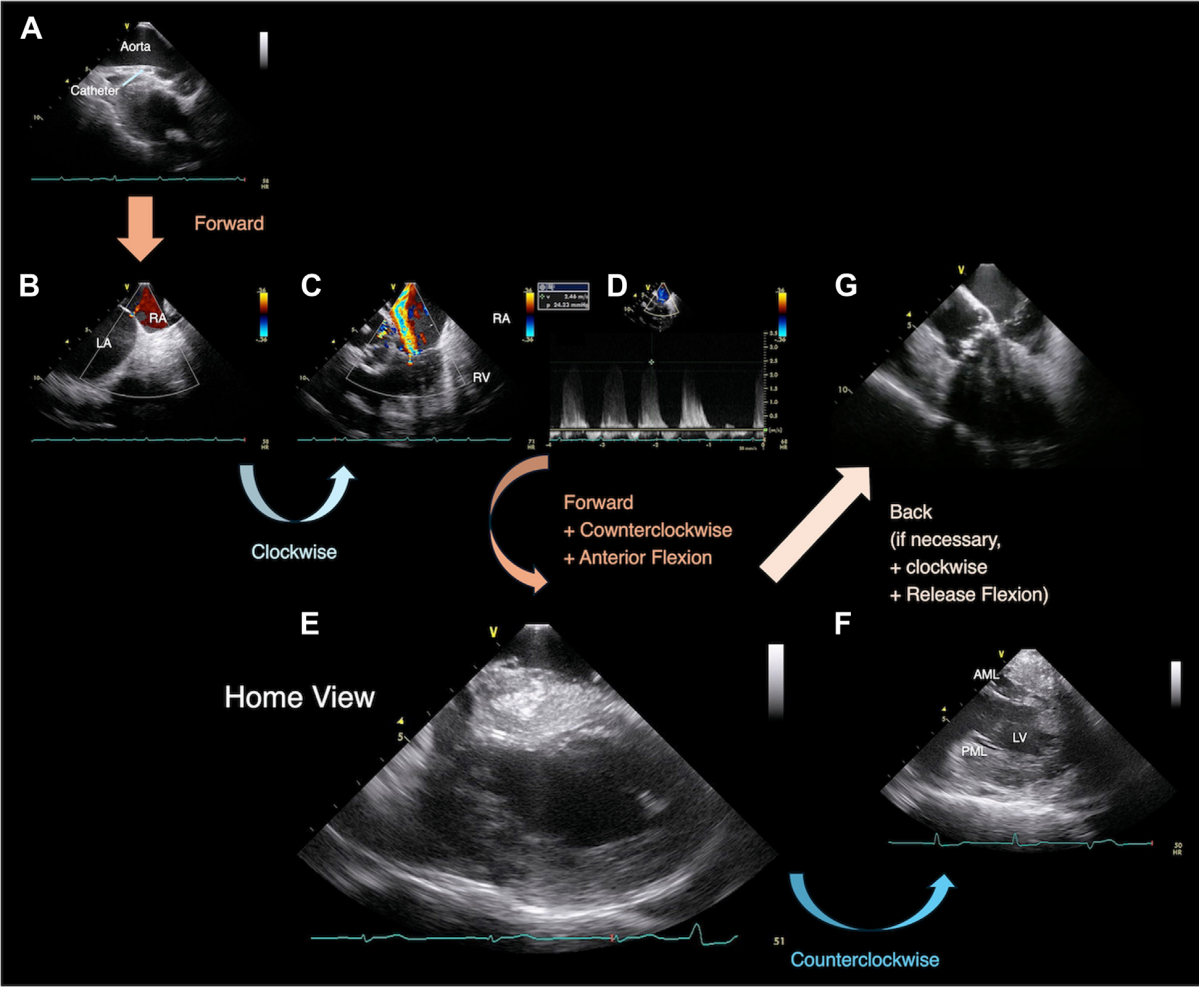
Multiple Views With Jugular Vein Intracardiac Echocardiography Identifying Complications or Hemodynamics (A) Long-axis ascending view. (B) Atrial septum view. There is small atrial septal defect with left-to-right shunt flow. (C) Long-axis tricuspid view. There is mild to moderate tricuspid regurgitation. (D) Pulse Doppler image of tricuspid regurgitation demonstrating the pressure gradient from the right ventricle (RV) to the right atrium (RA) of 24 mm Hg. (E) Long-axis left ventricular view. (F) Long-axis mitral valve view. (G) Long-axis aortic valve view. AML = anterior mitral leaflet; LA = left atrium; LV = left ventricle; PML = posterior mitral leaflet.ii.Atrial septum view (Figure 7B):•Advance the catheter a few centimeters farther (if necessary, add clockwise rotation).•Check for atrial septal defects or a patent foramen ovale.iii.Long-axis right ventricular (RV) view (Figure 7C):•Rotate the catheter clockwise.•Assess for preoperative tricuspid regurgitation and estimate RV systolic pressure (Figure 6D).iv.Long-axis LV view (home view) (Figure 7E):•Push forward into the right ventricle with anterior flexion and counterclockwise rotation.•Evaluate LV contraction, pericardial effusion, and wire position during the procedure.v.Long-axis mitral valve view (Figure 7F):•Rotate the catheter counterclockwise.•Assess the mitral valve complex.vi.Long-axis aortic valve view (Figure 7G):•Return the catheter clockwise and pull back to the right atrium.•Evaluate the aortic valve complex (if necessary).•Measure preprocedural aortic valve flow velocity (if necessary).c.Perform pretreatment TTE evaluation.4.Target identification and contrast agent injectiona.Administer analgesics.b.Perform coronary angiography.c.Monitor the contrast injection with ICE.d.Confirm the target location with ICE, and reassess if needed.5.Septal ablation and monitoringa.Continuously monitor with ICE during the ethanol injection.b.Evaluate for complications:i.Wall motion abnormalitiesii.Pericardial effusioniii.Mitral valve function changesiv.Ventricular septal rupturev.Other mechanical complications6.Postprocedure evaluation and managementa.Confirm full-cover ablation using ICE and TTE.i.Ensure ablation of the portion in contact with accelerated flow on color Doppler 2-dimensional imaging.^8^b.Remove the ICE catheter and arterial sheaths.c.Insert a temporary PM through the left internal JV.d.Remove the intraoperative PM and procedural venous sheaths.e.Transfer the patient to the coronary care unit.
